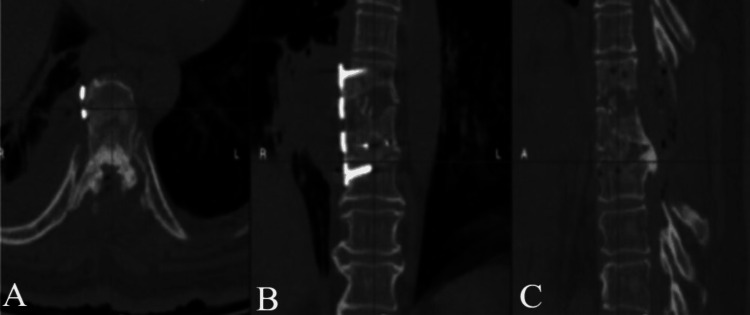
CT scan showing calcified extruded disc herniation at T7-T8, surgically treated with decompression via T7-T8 laminectomy in axial (A), coronal (B), and sagittal (C) plane.
